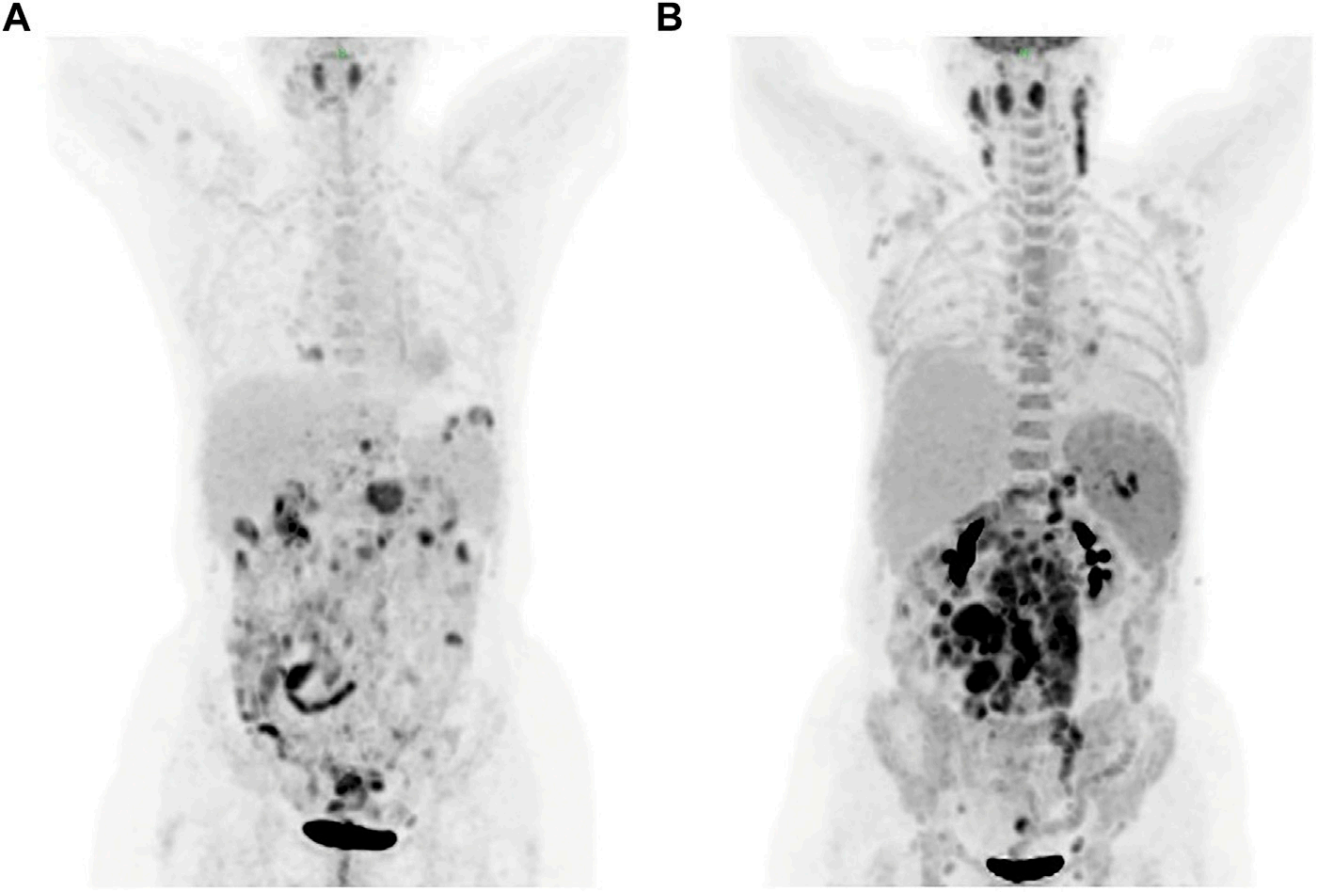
FDG-PET showed positive FDG uptake in multiple enlarged lymph nodes on 9 April (A) and 30 July (B), respectively. FDG-PET, positron-emission tomography with ^18^F-fluorodeoxyglucose.
